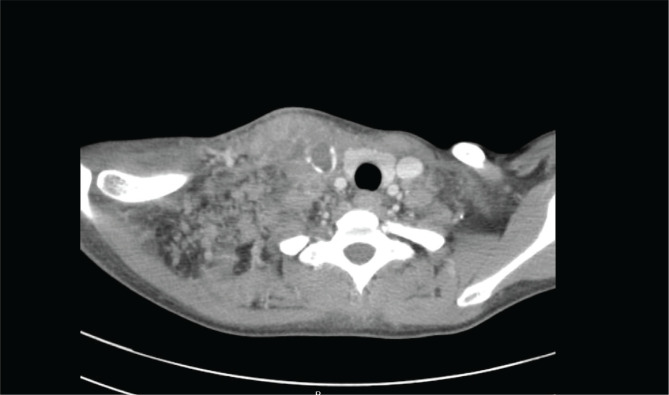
CT scan of the thorax (axial view) with extensive left supraclavicular lymphadenopathy.
